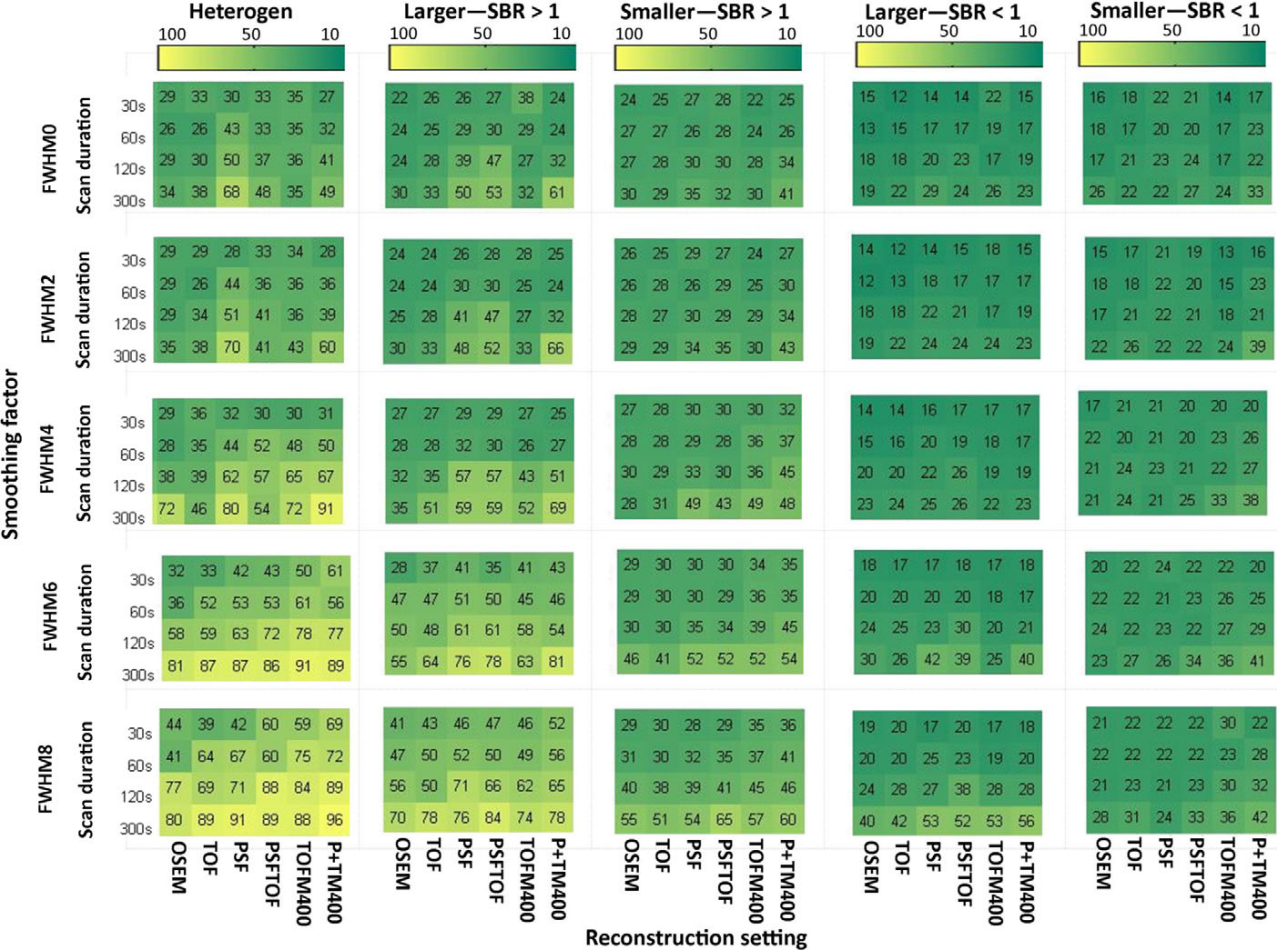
Percentage of all features discretized with FBN: Percentage of all features discretized with FBN and segmented based on CT exhibiting an ICC >0.8 for all studied settings and underlying data categories (from left to right: heterogeneous 3D prints, bigger spheres with high uptake, smaller spheres with high uptake, bigger spheres with low uptake, and smaller spheres with low uptake). TOFM400/P+TM400: TOF/PSF+TOF reconstruction with matrix size 400 × 400. [Color figure can be viewed at wileyonlinelibrary.com]
